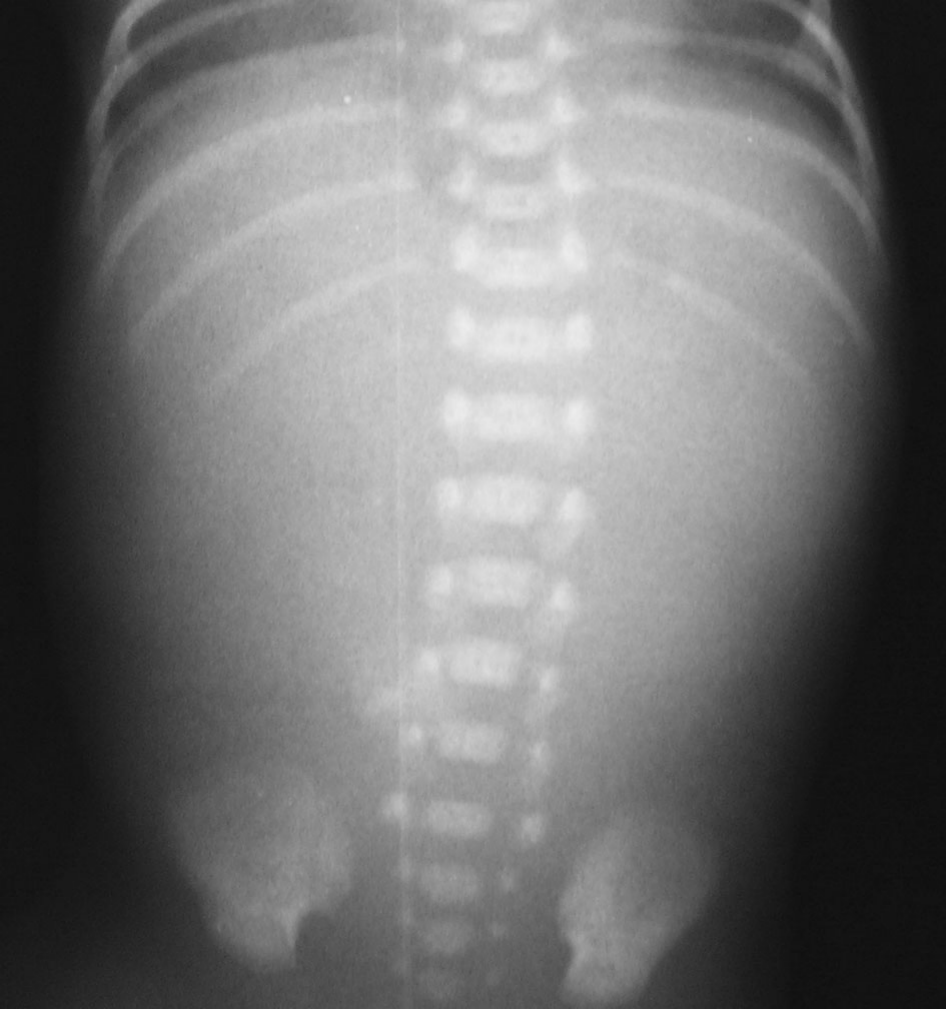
Radiograph demonstrates a gasless abdomen without findings of pneumoperitoneum; these findings cannot be classified according to DASS. However, according to the literature, it is the sign of occult perforation and advancing peritonitis
